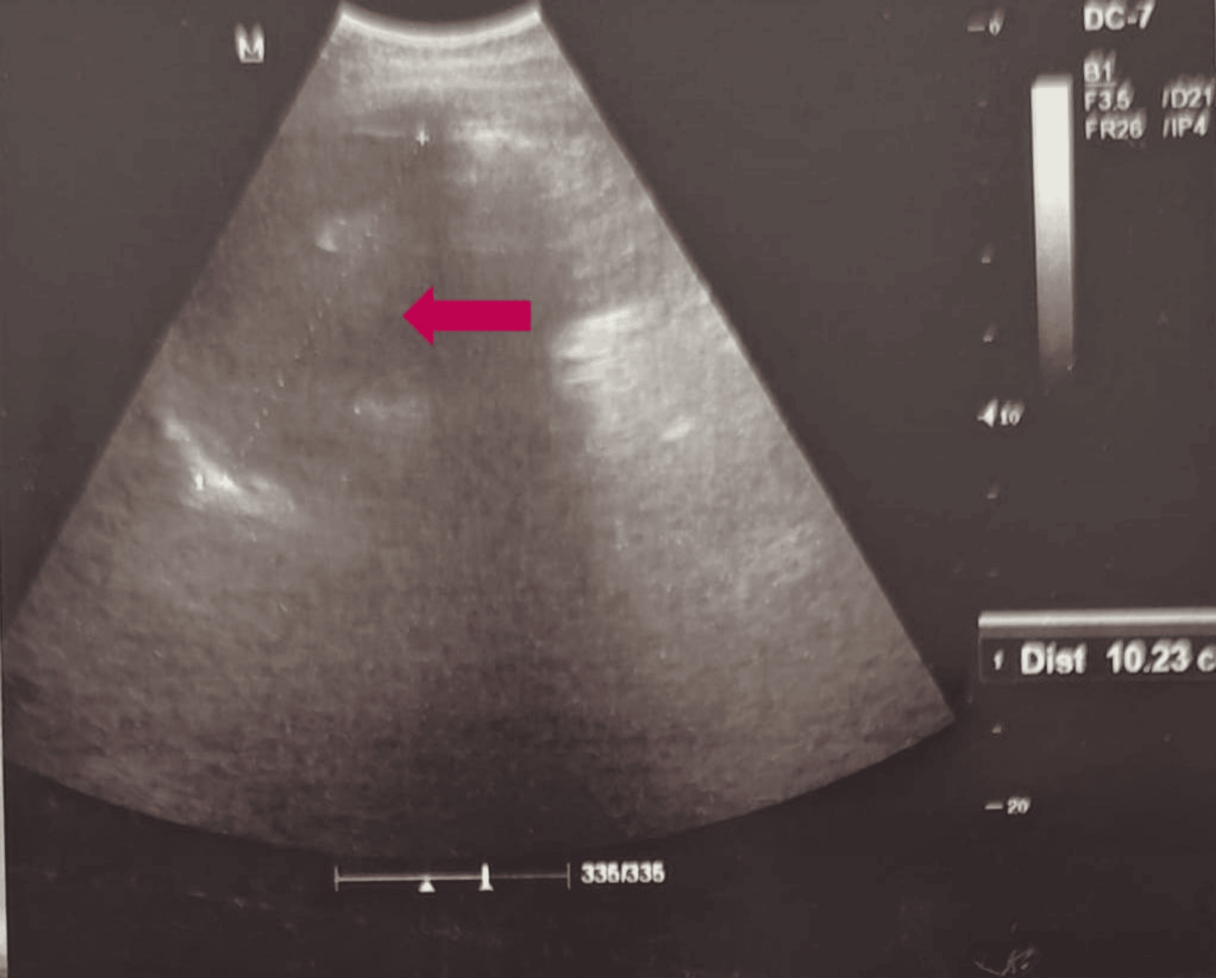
USG of the abdomen showing a heteroechoic lesion (red arrow) located posterior to the umbilicus USG: ultrasonogram
